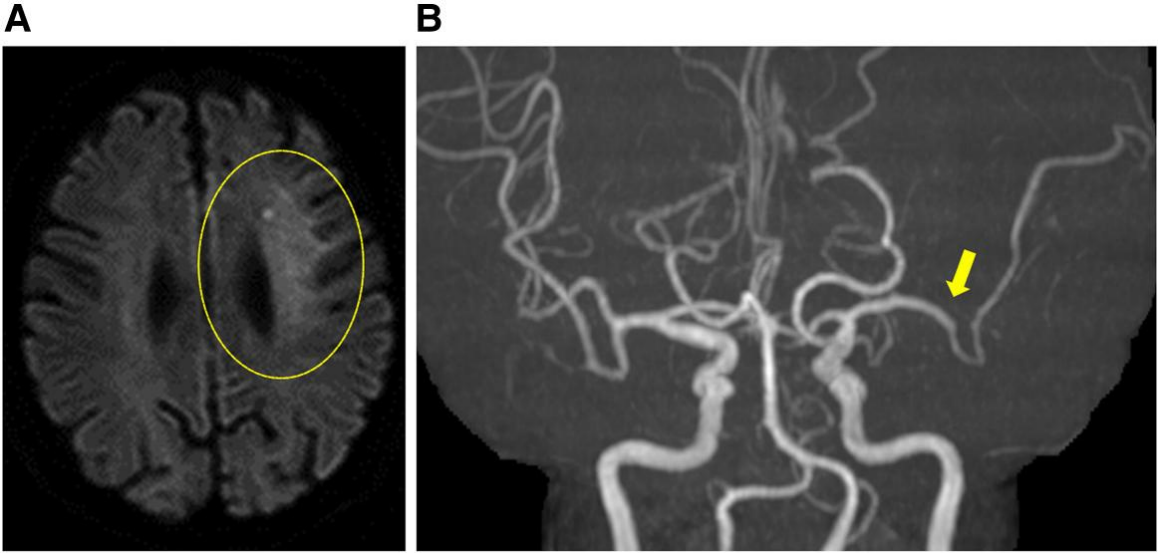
(*A*) Brain magnetic resonance imaging with diffusion-weighted imaging showed an acute infarction in the left frontal lobe (circle). (*B*) Magnetic resonance angiography showed an occlusion of the anterior branch of the left middle cerebral artery (arrow).
